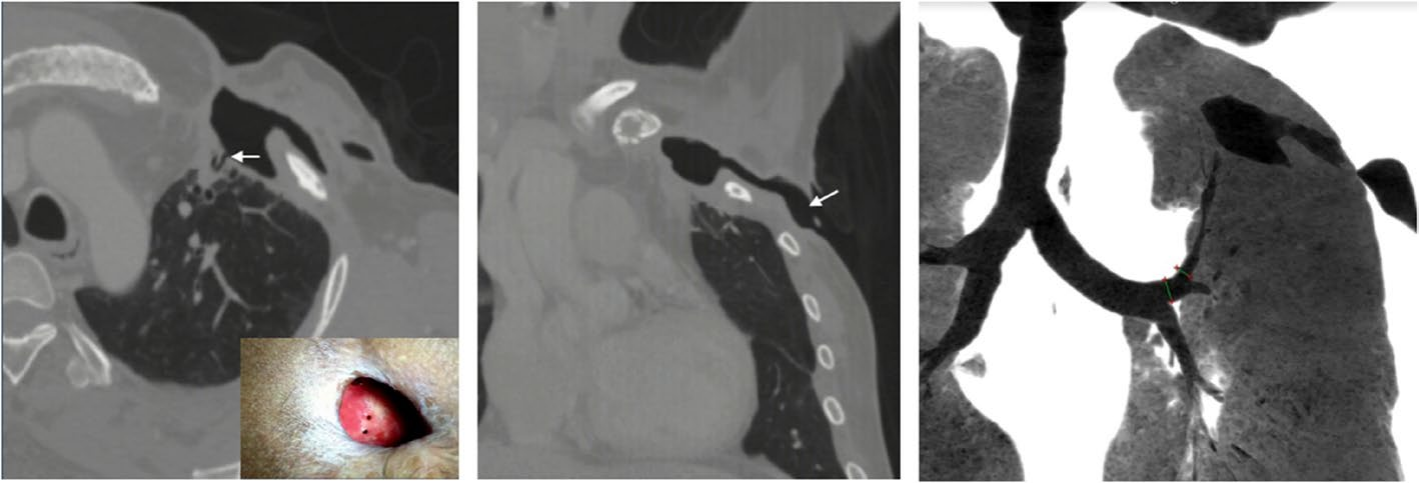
a Axial computed tomography (CT) lung window showing the peripheral bronchopleural fistula (arrow). Inset depicts the skin site over the patient's left chest with two openings. b Coronal CT lung window showing the pleural to cutaneous component(arrow) of the fistula. c Coronal CT Minimum intensity projection image showing the apicoposterior segment bronchus extending to the pleural surface and further to the skin
